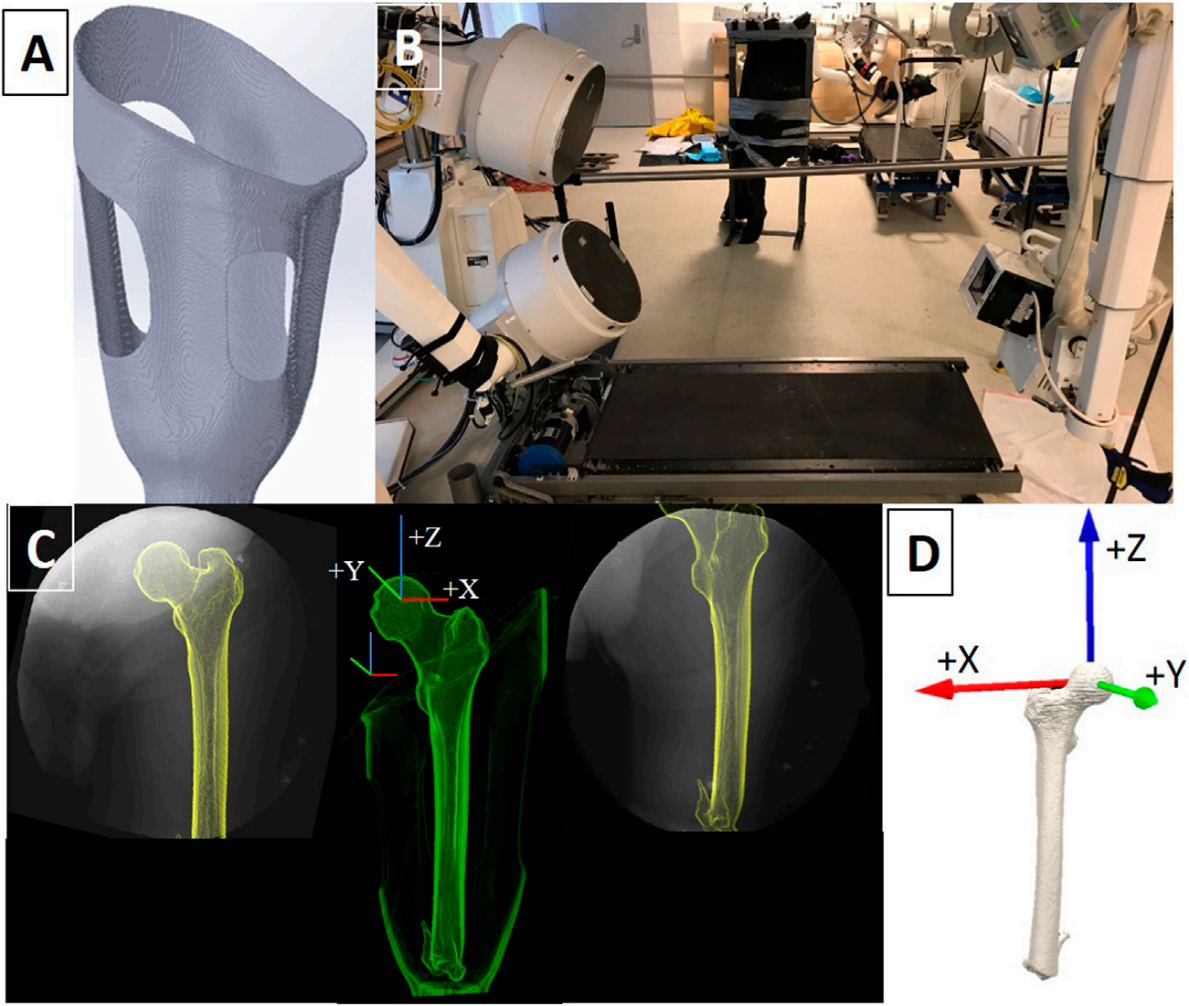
(A) Example of a digitally rendered transfemoral CRS socket with longitudinal depressions (struts) and open windows to accommodate the displaced tissue. (B) The DSX system was set up to record dynamic x-ray sequences in a 60° anteroposterior orientation as participants walked on the treadmill. (C) X-ray field of view images of the residual limb-socket complex from both x-ray views and the corresponding fusion of x-ray and CT imaging into a 3D entity. Femur and socket coordinate systems are displayed. (D) The anatomical coordinate system assigned to the residual femur was located at the center of the femoral head.
